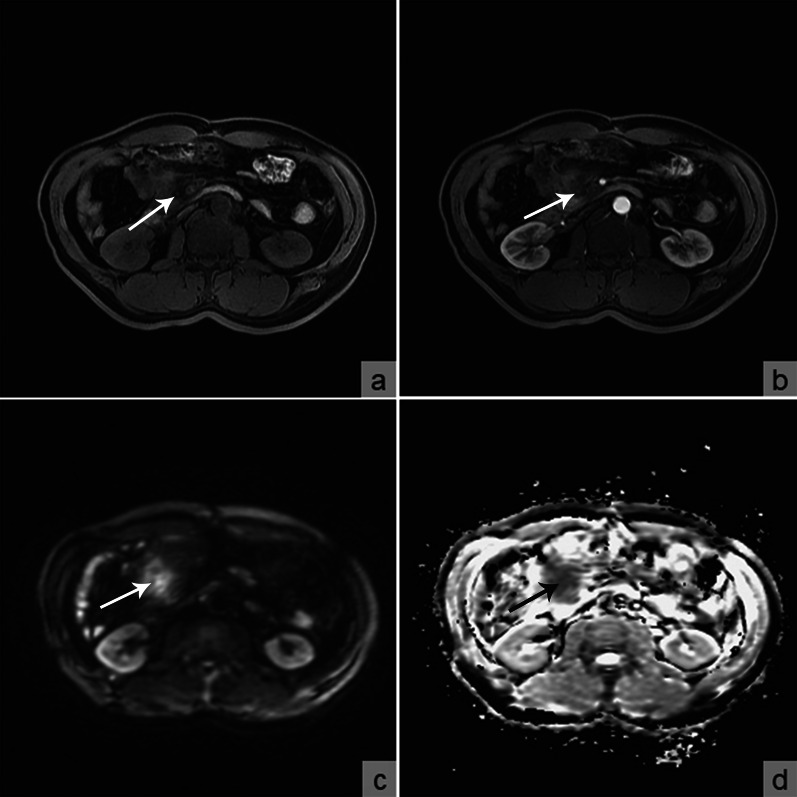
Abdominal MRI revealed a heterogeneous infiltrative soft tissue lesion near the previous ileum–colon anastomosis exhibiting a hypointensity on precontrast T2-weighted imaging (white arrow); b delayed and moderate enhancement on postcontrast T2-weighted imaging (white arrow); c hyperintensity on diffusion-weighted imaging (white arrow), and d water restriction on the ADC map (black arrow)
